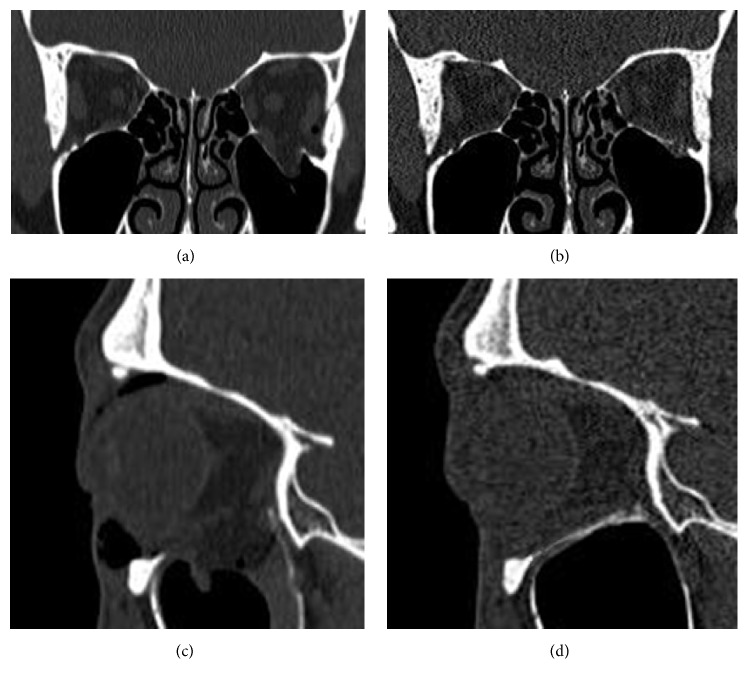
Pre- and postoperative CT views of a 38-year-old male patient who had a large orbital defect reconstructed with a resorbable plate and artificial bone substitute. Coronal view of preoperative CT showed a left-sided orbital floor defect of 4.2 cm^2^ with inferior rectus muscle displacement (a). This patient had discomfort with upper gaze, but no extraocular muscle limitation. Eighteen-month postoperative CT scan (coronal view) showed a reconstructed orbital floor with thin bone compared with the opposite side (b). The sagittal plane of the pre- and postoperative CT images revealed a nearly anatomically reconstructed orbital floor with no orbital volume discrepancy (c, d).
